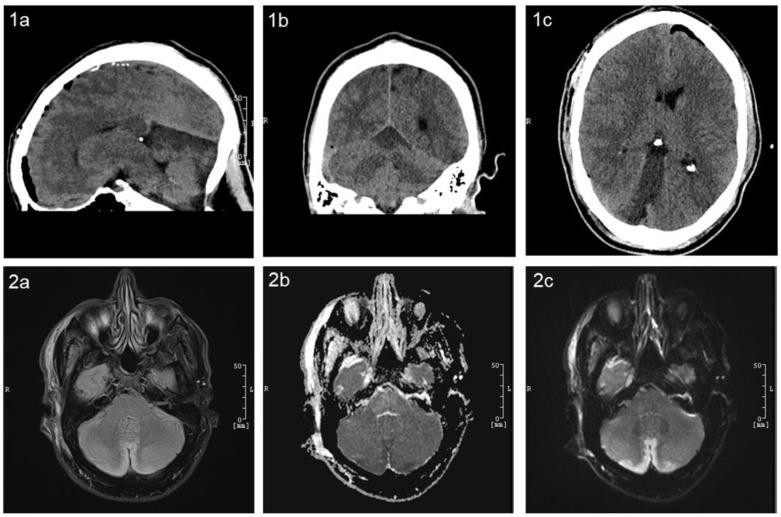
Non-contrast CT and MRI of the brain showing a hypodense area in the superior cerebellum, consistent with an infarction in the territory of the superior cerebellar artery (SCA). This finding correlates with the patient’s perioperative neurological symptoms. Panels (1a–c) show the postoperative CT scan in sagittal, coronal, and axial views. Panel (2a) displays the postoperative brain MRI in T2-FLAIR sequences, while panel (2b) presents diffusion-weighted imaging with apparent diffusion coefficient mapping sequences and (2c) present standard diffusion-weighted imaging sequences.
